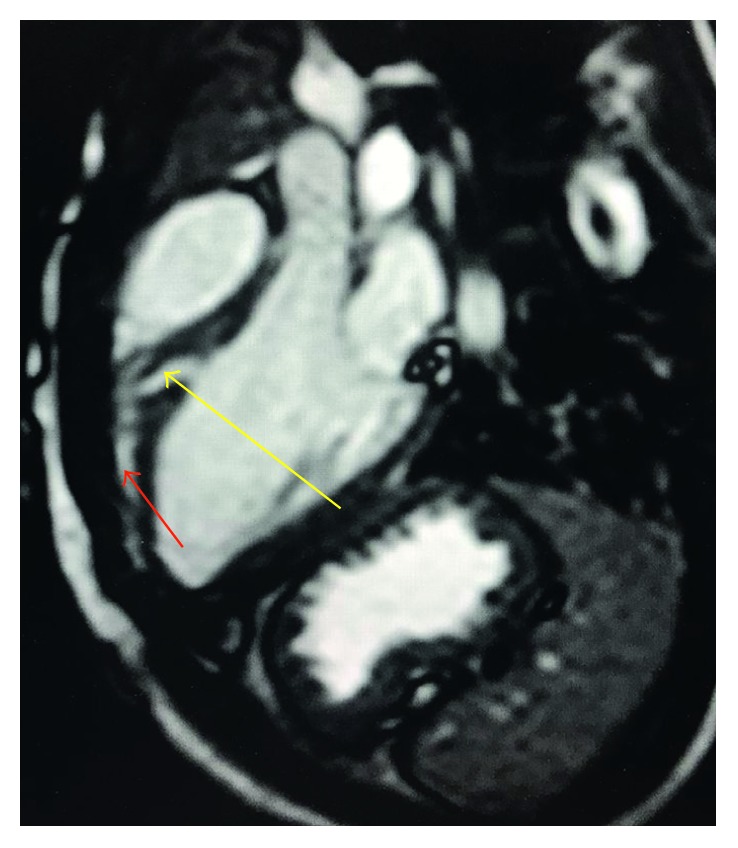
Cardiac MRI (left ventricular outflow tract view) showing a small muscular VSD (yellow arrow) covered by hypertrophied trabeculations on the RV side (red arrow).
